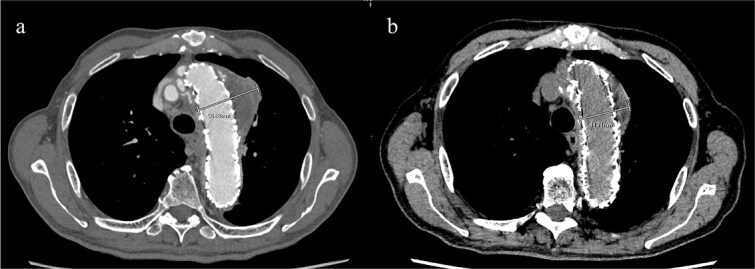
(a) Contrast-enhanced CT performed soon after TEVAR showing an aneurysm diameter of 56 mm. (b) Two years later, contrast-enhanced CT showing a reduction in the aneurysm diameter to 44 mm.
